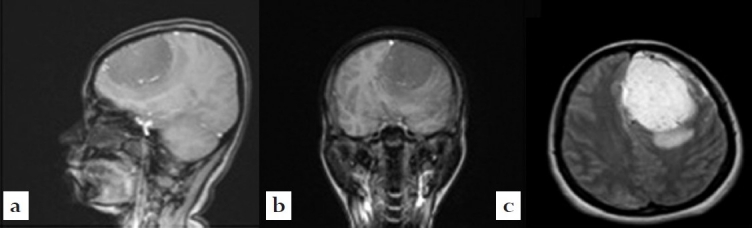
Magnetic resonance imaging of the brain. (a) Sagittal view (T1 weighted); (b) coronal view (T1 weighted); (c) axial view (FLAIR). Imaging demonstrated a 4.9 × 7.2 × 4.8 cm extraaxial mass with surrounding edema and resulting mass effect causing a 14mm rightward shift of the midline structures. There was compression of the frontal horn of the lateral ventricle and the third ventricle, deformity of the midbrain on the left and moderate dilatation of the lateral ventricle. The mass had signal voids which were likely representing vascular flow. There was surrounding vasogenic edema extending throughout the adjacent left frontal white matter.
